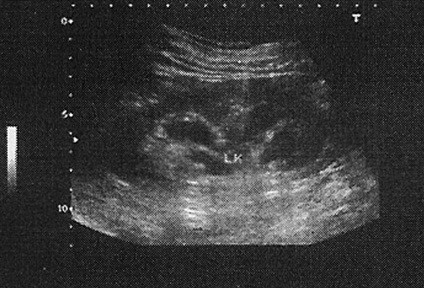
Hydronephrosis appearance in sonography.
